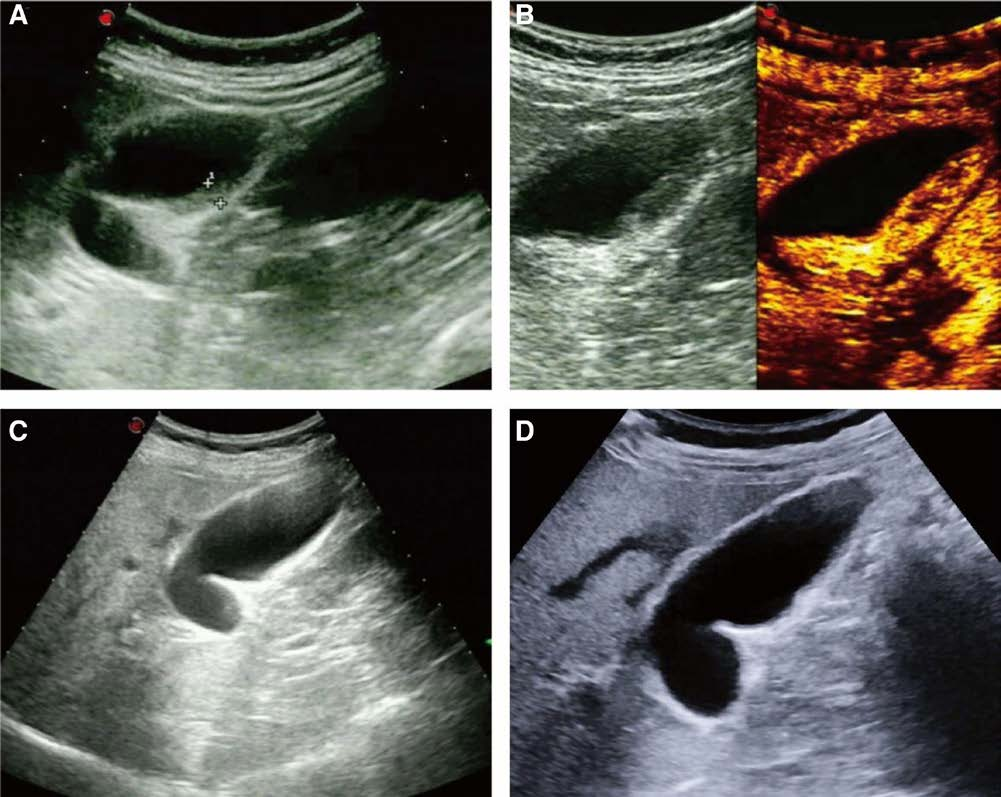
Postoperative follow-up ultrasound images of patients. (A) 1 week after surgery, the gallbladder wall was slightly rough, with local thickening up to 5.8 mm, and the internal echo was not uniform. (B) Postoperative 1 week, CEUS showed good continuity of the gallbladder wall and uneven high enhancement in the thickened area of the gallbladder wall. (C, D) 1 month and 3 months after surgery, ultrasound showed smooth gallbladder wall and no obvious abnormal echo in the cavity. CEUS = contrast-enhanced ultrasound.
